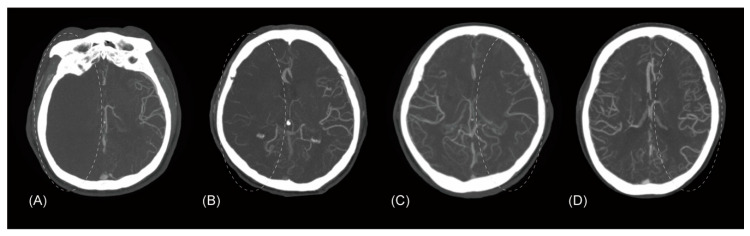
Assessment of leptomeningeal collaterals according to collateral assessment method 1 (Tan et al.’s method). Four computed tomography angiography maximum intensity projection axial images from different patients showing leptomeningeal collaterals. (A) Absent collateral supply to the occluded middle cerebral artery territory (circle), compared to the contralateral normal side. (B) Collateral supply of greater than 0% but less than or equal to 50%. (C) Collateral supply of greater than 50% but less than 100%. (D) Complete collateral supply of 100%.
